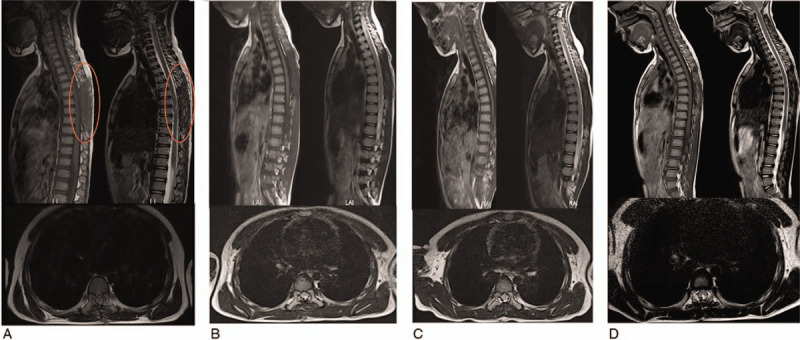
The magnetic resonance imagings at different timepoints after operation. (A) 1 m, (B) 3 m, (C) 6 m, (D) 12 m. The area shown in the red circle is the operation area.
